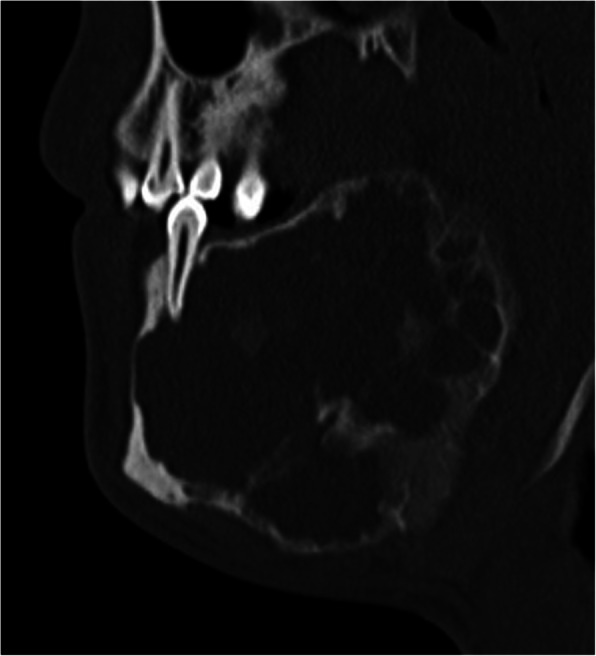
Computed tomography shows a mainly cystic lesion; the tumour proper is the solid area in the labial aspect of the lower part (arrow)
